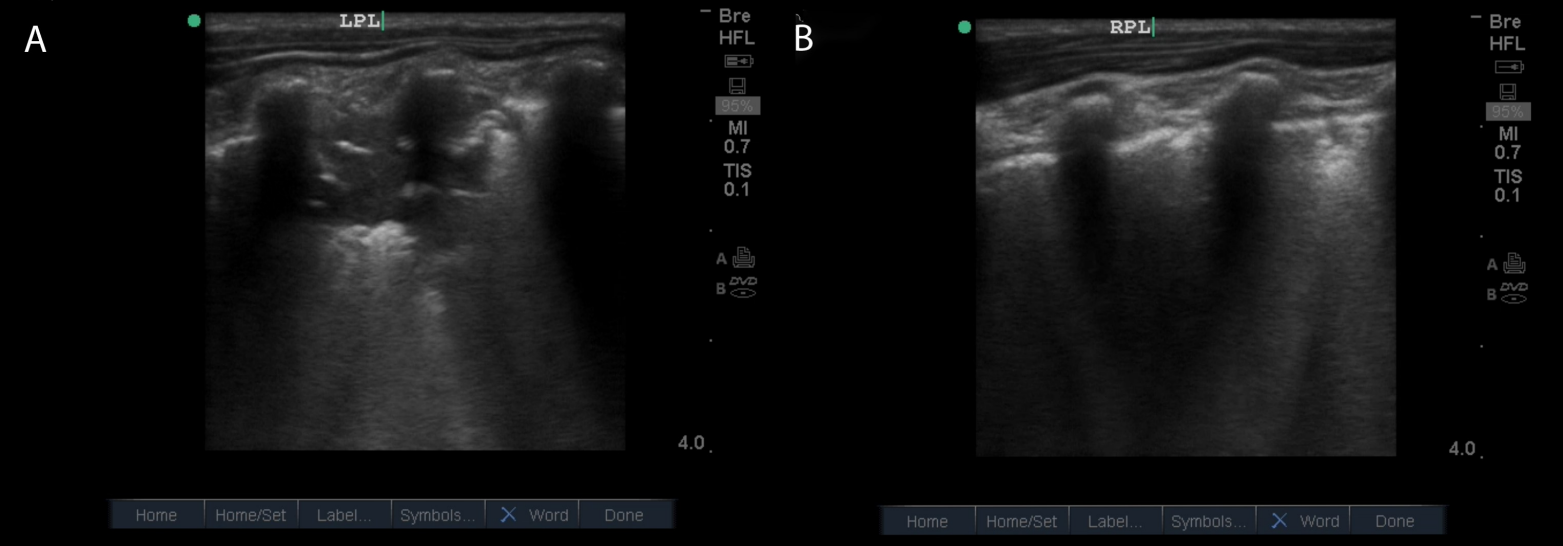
Lung point of care ultrasound (POCUS) showing pneumonia. A. Longitudinal views of the left posterior lung (LPL) showing large lobar consolidation. B. Longitudinal views of right posterior lung (RPL) showing subpleural consolidation with confluent B-lines.
